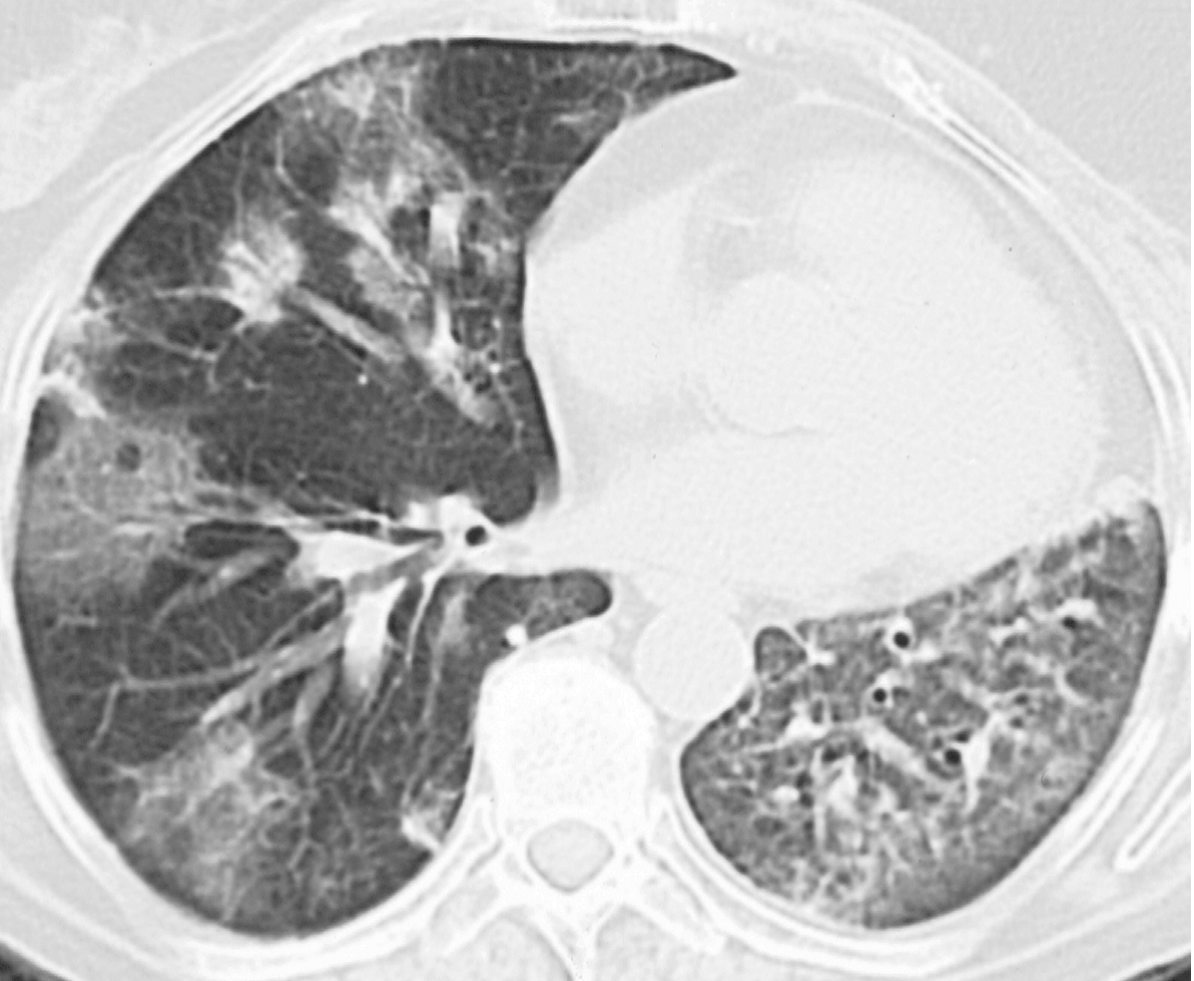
CT shows patchy areas in the left lung and patchy areas in the middle lobe of the right lung.
